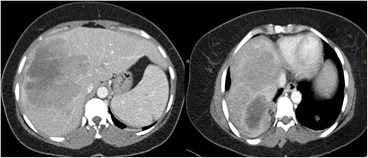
Transaxial images of the contrast-enhanced CT scan of the abdomen at the portal venous phase shows a multifocal, ill-defined, and relatively hypodense hepatic lesion that involves the right and left hepatic lobes and surrounds an enhanced pseudocapsule. There are multiple subcapsular undulated margins, which are suggestive of subcapsular retraction. The lesions show mass effects on the intrahepatic portion of the IVC with significant narrowing and medial deviation. The indistinct appearance of the middle hepatic vein and the distal branches of the portal vein suggest that HCC invaded these areas
